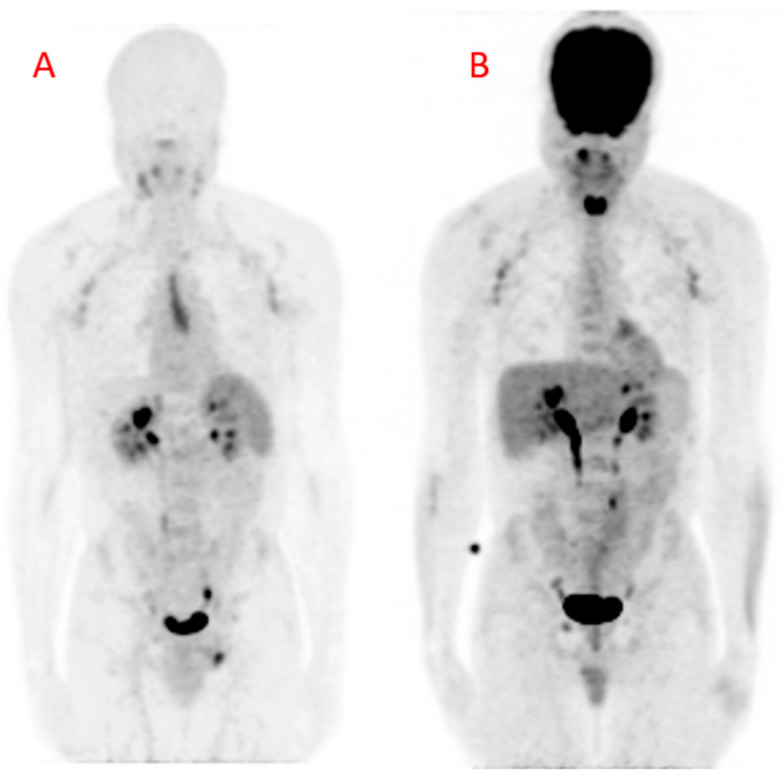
Maximum intensity projection (MIP) images obtained from (A) Gallium-68 pentixafor positron emission tomography ([^68^Ga]Ga-pentixafor PET) and (B) fluorine-18 fluorodeoxyglucose positron emission tomography (^18^[F]FDG PET) in a 42-year-old HIV-infected male. There are multiple mildly avid axillary lymphadenopathies and a urinary route of excretion of tracers on both images. The [^68^Ga]Ga-pentixafor PET image (A) shows physiologic tracer uptake in the spleen. The midline area of linear tracer uptake is localized to the thymus, likely related to inflammation associated with combination antiretroviral therapy (cART)-induced immune restoration. The [^18^F]FDG PET image (B) shows intense tracer uptake in the brain and the nasopharynx. There is also intense [^18^F]FDG in the muscles of phonation because the patient was talking during the period of uptake. This intense uptake can cause photon spill-over into the carotids during tracer uptake quantification. The patient arrived for the [^18^F]FDG PET/CT carrying a bag causing the diffuse [^18^F]FDG uptake in the lateral aspects of the forearms, more pronounced on the left. There is only minimal myocardial [^18^F]FDG uptake due to prolonged fasting in this patient.
